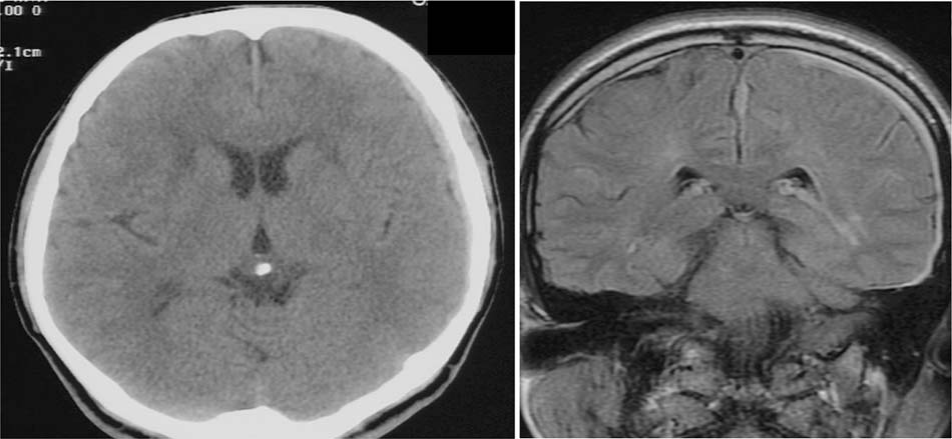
CT (left) axial image of the brain and MRI (right) fluid-attenuated inversion recovery coronal image from the referring hospital. SDH is visible on both images, without evidence of SAH. SDH is also visible in the interhemispheric fissure on MRI.
